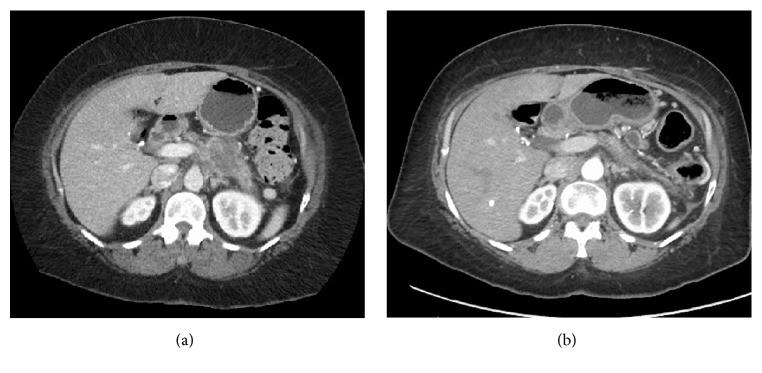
(a) Axial CT demonstrating large mass in body/tail of pancreas before neoadjuvant therapy. (b) Axial CT demonstrating tumor response after neoadjuvant therapy.
